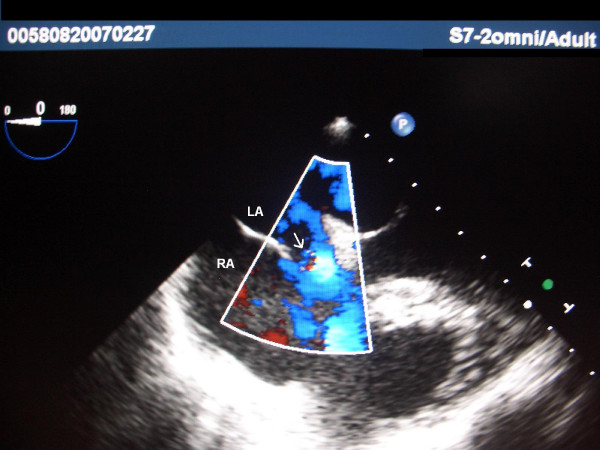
TEE image 1 of the first patient. Color Doppler imaging depicts the interatrial shunting (arrow). LA – Left Atrium; RA – Right Atrium.
